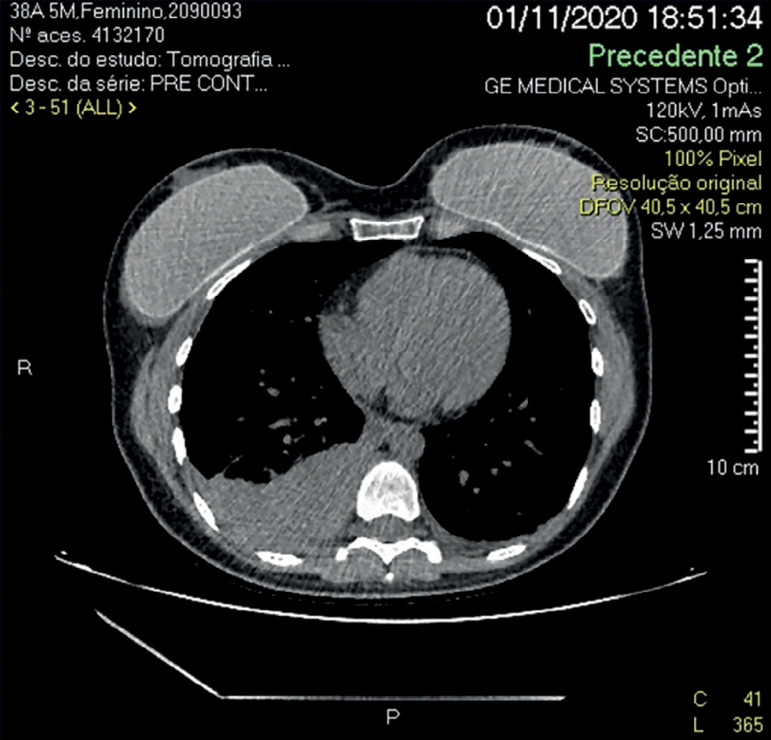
Computed tomography scan showing hemothorax, 10 hours after oocyte retrieval.
